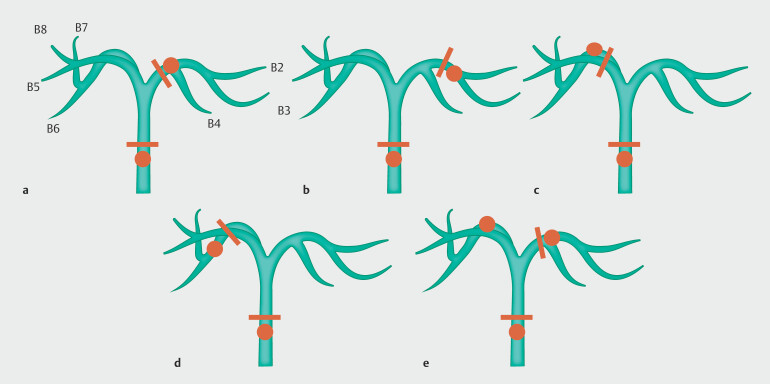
The limit of ductal transection and the biopsy sites in mapping biopsy (MB) based on the planned surgical procedure. The limit of ductal transection is shown as a black line and the MB sites are indicated by black circles. These were determined based on the planned surgical procedure from the multi-detector computed tomography images prior to endoscopic retrograde cholangiopancreatography. The limitation line on the duodenal side corresponds to the upper edge of the pancreatic head, and the MB site on the duodenal side is the distal bile duct. The MB sites were as follows.
